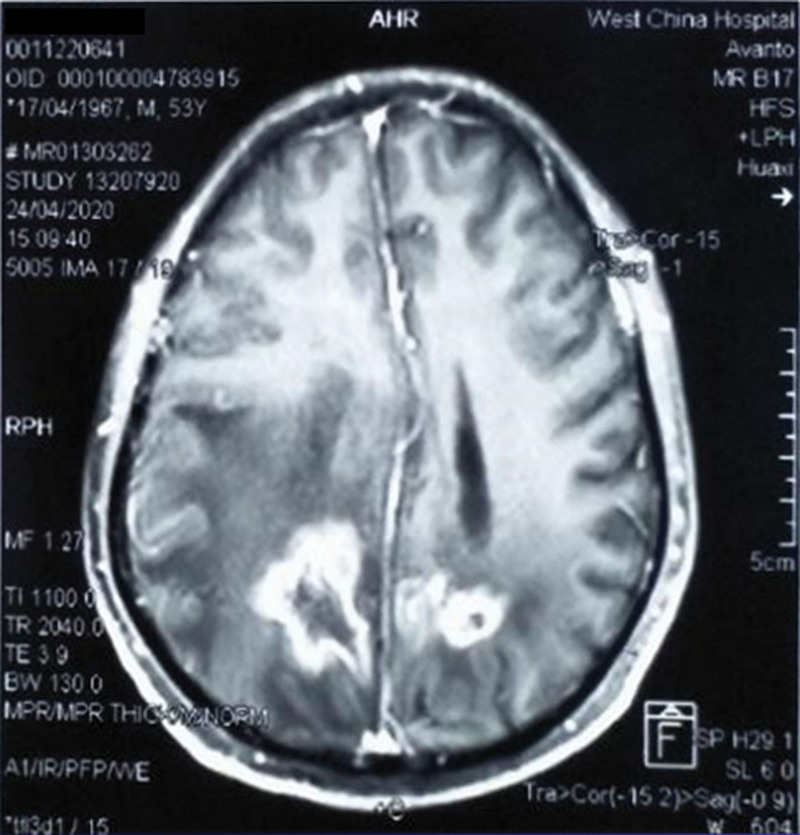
In April 2020, MRI indicated the larger section of a target lesion in the parietal lobe at 5.1 × 4.1 cm in size. MRI = magnetic resonance imaging.
